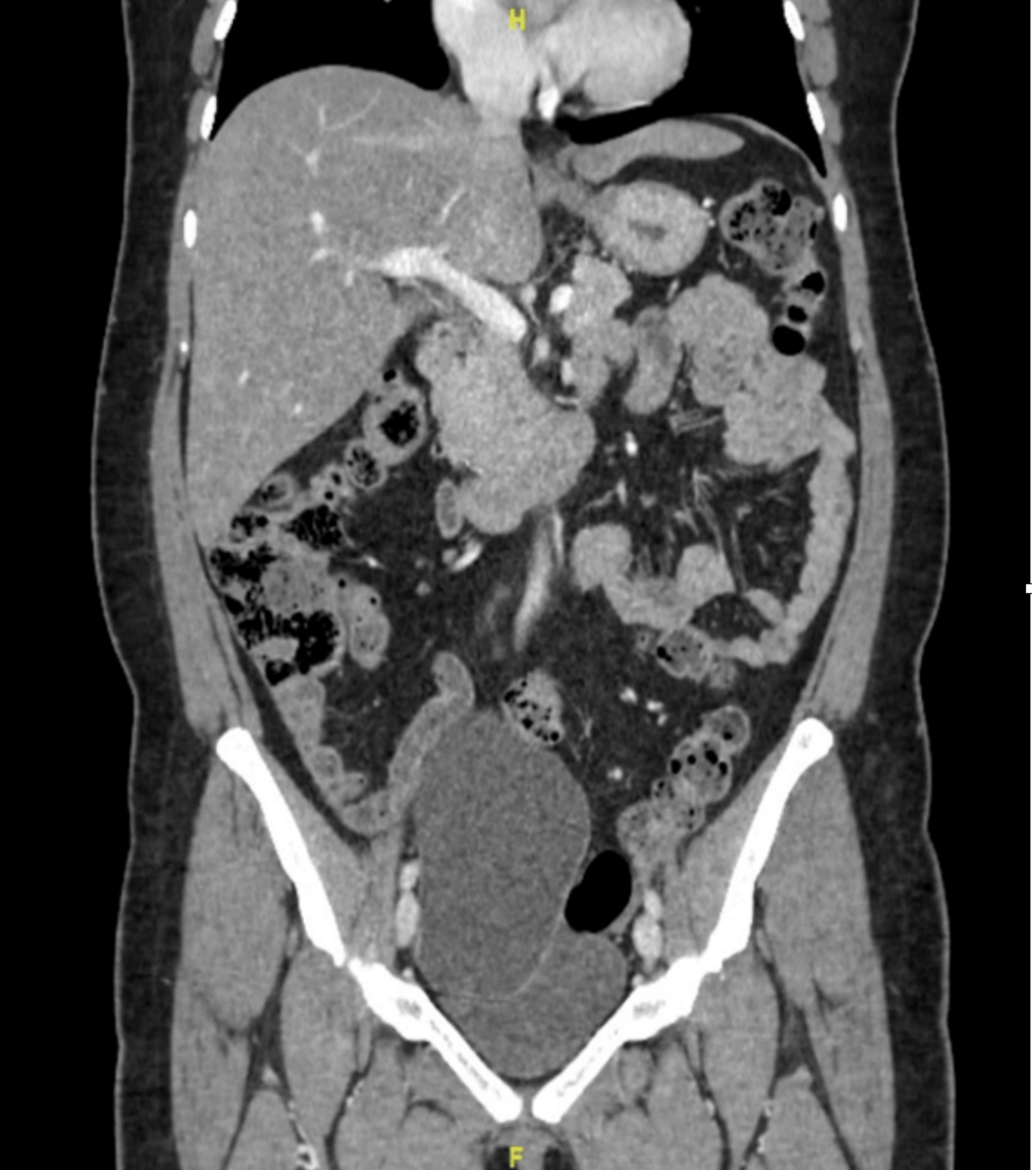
CT imaging showing a left sided adnexal cyst
